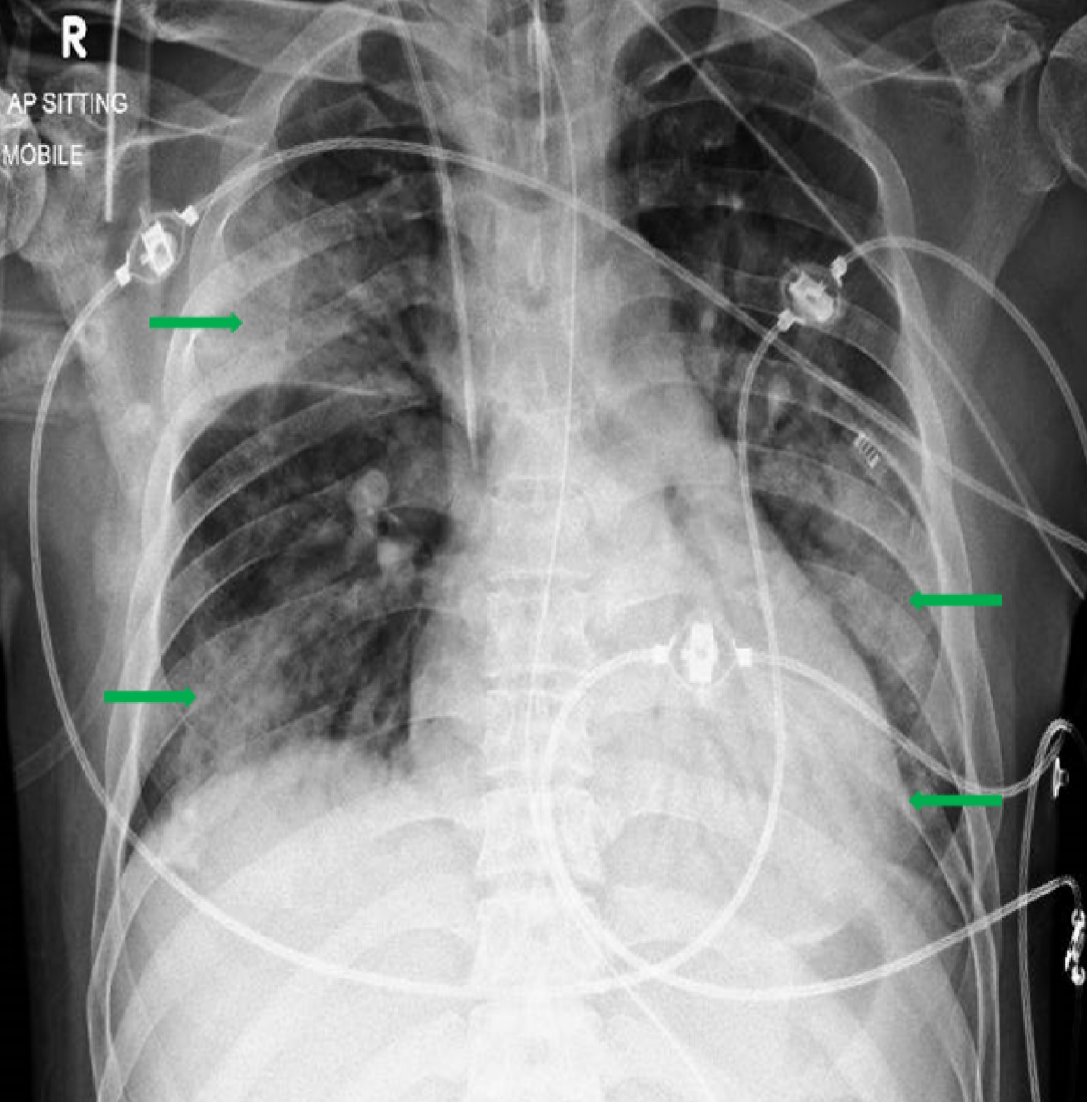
Chest radiograph on admission to the emergency department Extensive bilateral airspace changes (green arrows), involving the right upper and left middle zones and both lung bases
